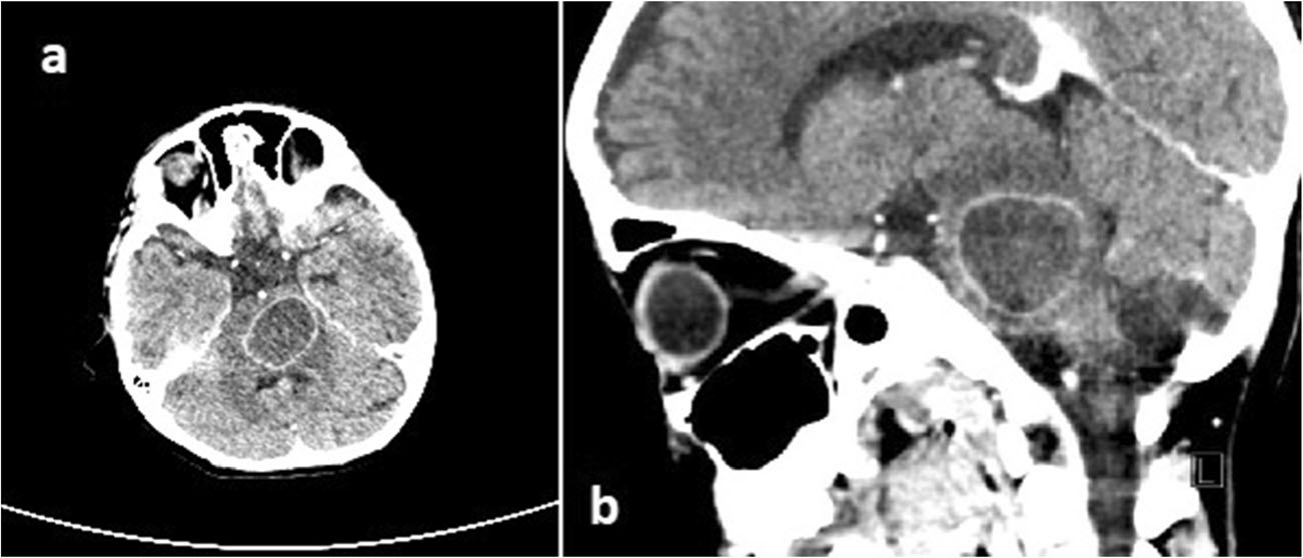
CT with contrast a axial and b sagittal reconstruction showing enlargement of the abscess (performed before biopsy)
